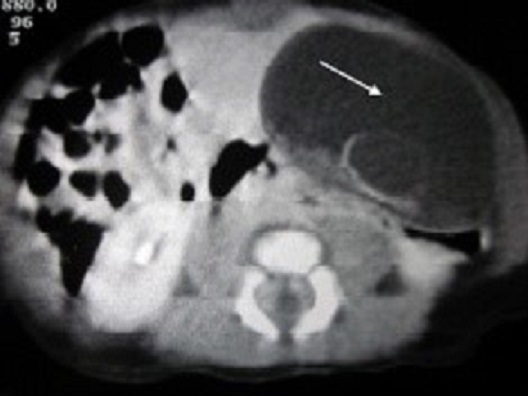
CT scan of the abdomen: voluminous compressing fluid-filled mass with a thin wall and a dense content
